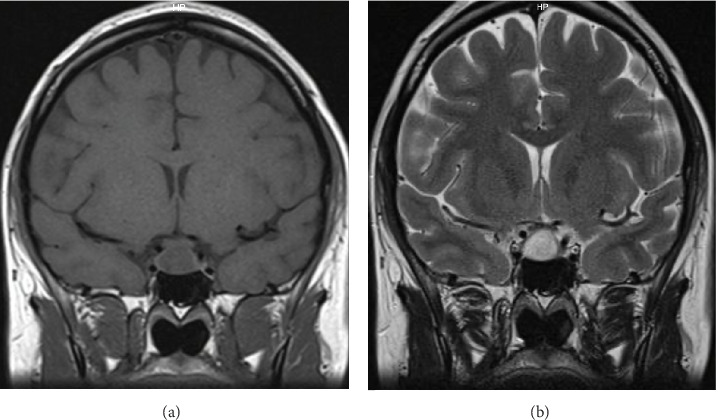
MRI, (A) T1 coronal view without contrast and (B) T2. An increase in tumor size is observed, measuring 12 × 19 × 11 mm. with intratumoral bleeding.
